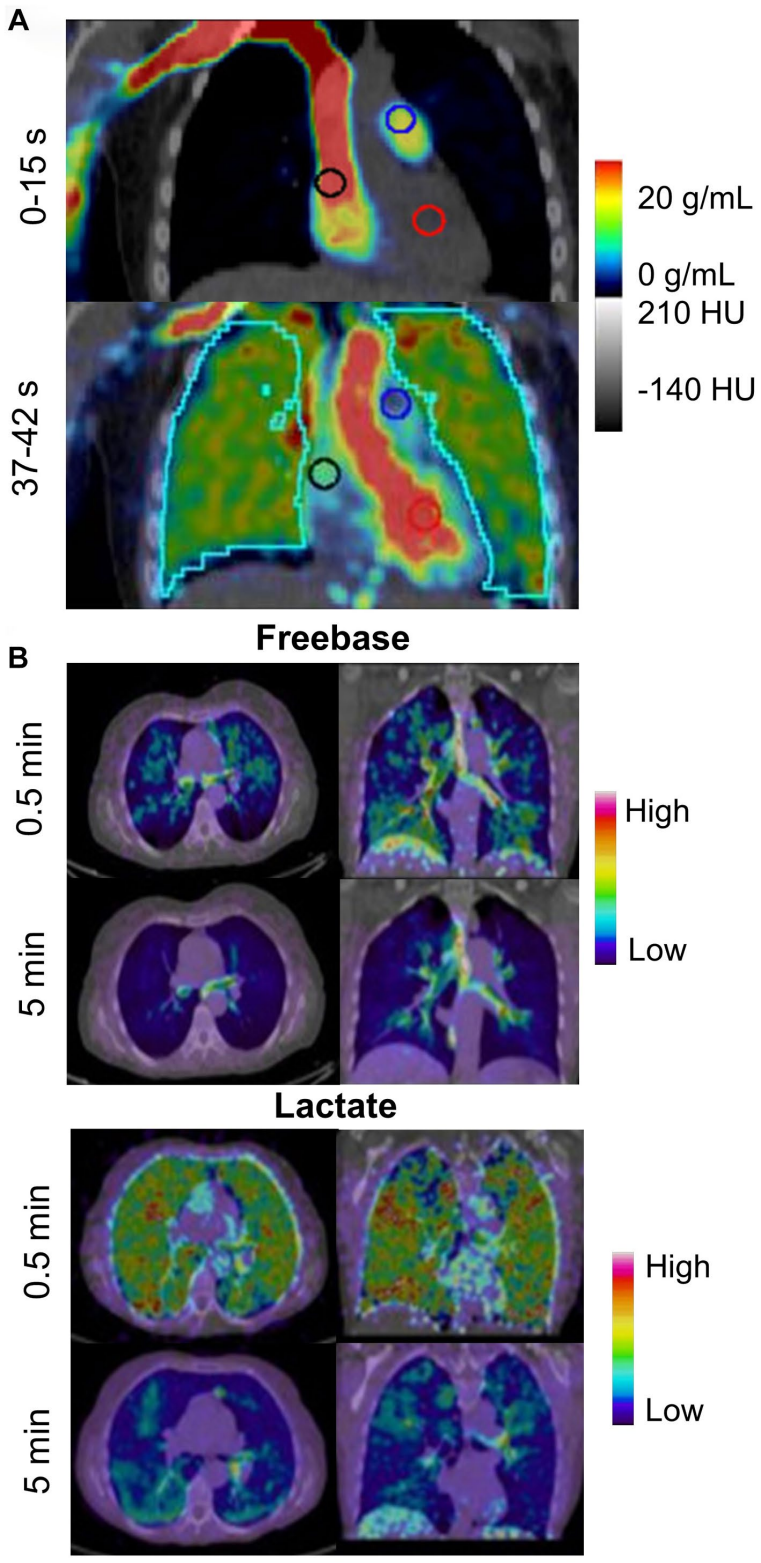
PET imaging tracking metabolic activity in e-cigarette users and smokers. (A) Oronal PET/CT ^18^F-NOS in an e-cigarette user (unspecified active ingredient). Uptake is shown 0–15 s and 37–42 s after injection of ^18^F-NOS to quantify oxidative stress and inflammation in the lungs. Adapted from Wetherill et al. (109) under Creative Commons License. (B) Representative distribution of ^11^C-nicotine freebase and lactate in lungs at 0.5 and 5 min after inhalation of the tagged nicotine salts through an e-cigarette in a healthy adult smoker. Adapted from Wall et al. (92) under Creative Commons License.
